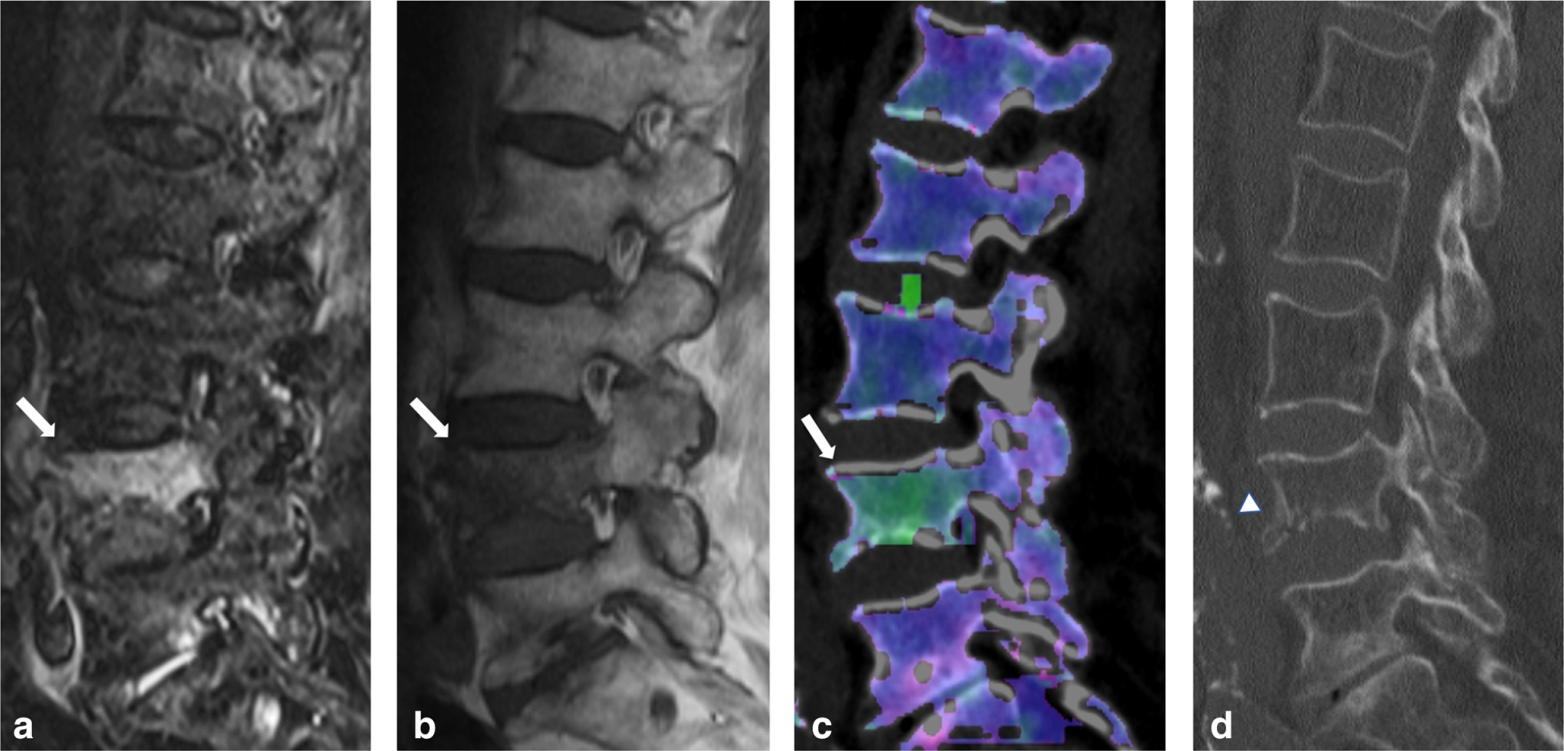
Eighty-seven-year-old woman presenting with acute spinal trauma due to a domestic fall. a Sagittal turbo inversion recovery magnitude (TIRM)–magnetic resonance imaging (MRI) series, b spin-echo (SE) T1-weighted MRI series, and c dual-energy computed tomography (DECT)–virtual non-calcium (VNCa) reconstructions showing bone marrow edema (BME) in all four quadrants of L4 (*arrow*). All readers were concordant in assessing BME presence (score 3 = distinct BME) and extent (score 4 = all quadrants) on both techniques. d In addition, sagittal conventional grayscale DECT images allowed for detection of an acute slightly dislocated fracture of the ventral ground plate of L1 (*arrowhead*) in terms of a tear drop fracture with potentially associated instability by all readers in this study. Confidence in depicting fracture lines was rated as intermediate (score 2) and high (score 3) by 5/5 readers on MR and DECT image series, respectively
